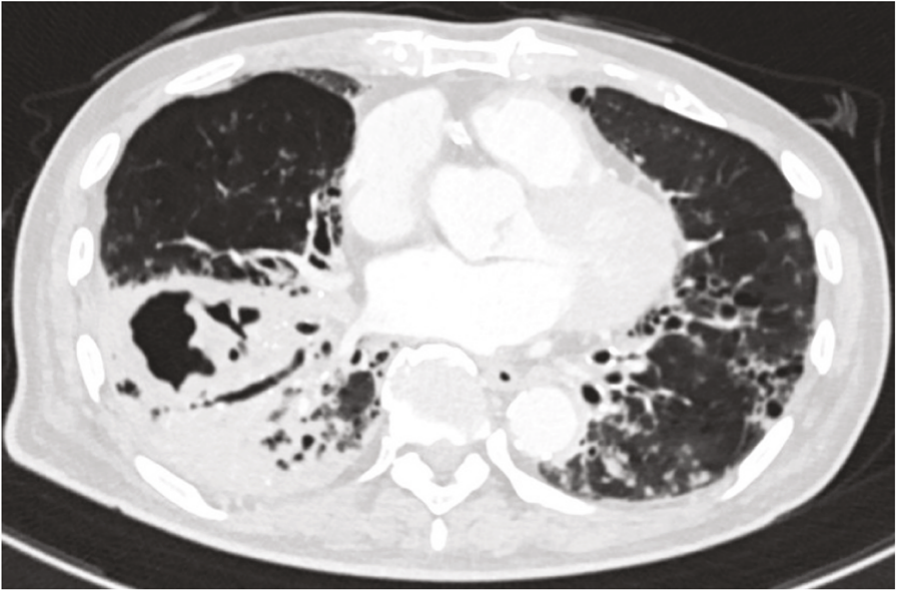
The chest CT lung window imaging shows bilateral bronchiectasis and a large bulla with surrounding consolidation in the right lower lobe
